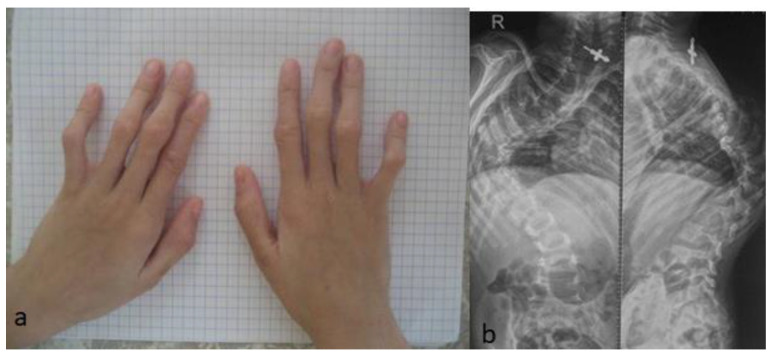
Arachnodactyly associated with camptodactyly of the 5th. Fingers, in a 4-year-old girl (a); AP and lateral spine radiographs showed kyphoscoliosis (Cobbs angle of 80°) (b).
